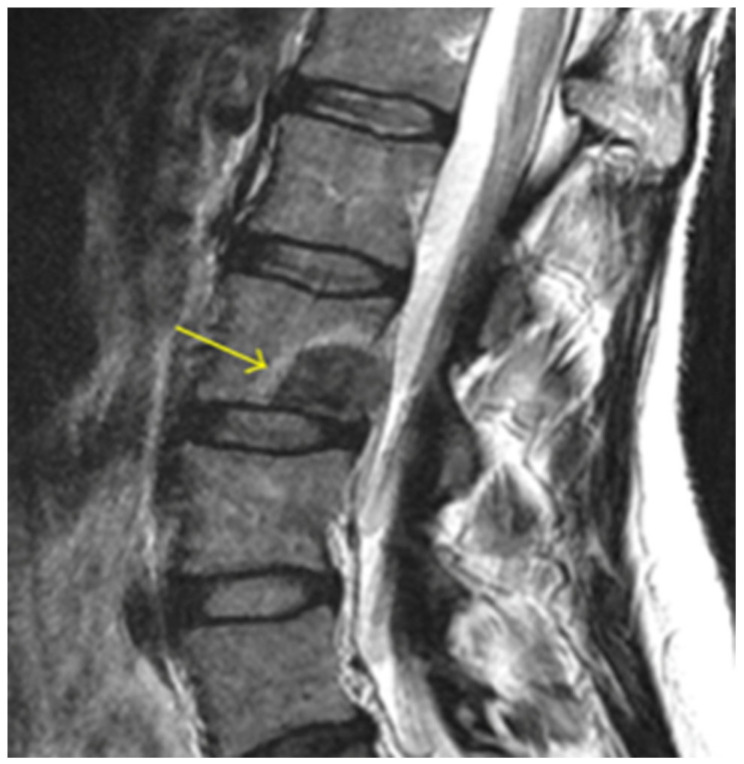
Lumbar spine magnetic resonance image (MRI) with an arrow pointing at the posterior–inferior edge of the vertebral body, highlighting a vertebral body tumor.
